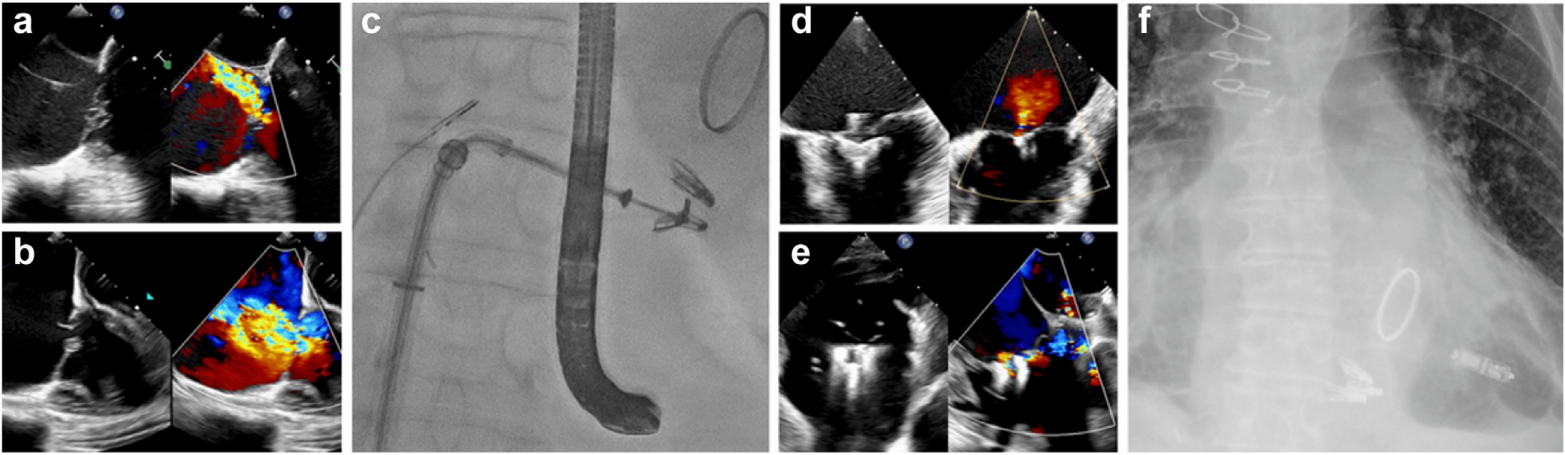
A case representative of the challenges presented with CIED and tricuspid regurgitation. A 91-year-old male with atrial fibrillation, a mechanical mitral valve, and a pacemaker with a single right ventricular lead developed severe tricuspid regurgitation and congestive hepatopathy-related cirrhosis. He was referred for lead extraction, placement of a leadless pacemaker, and ultimately TEER of the tricuspid valve with three MitraClip devices. (a) Pre-extraction TEE revealing severe TR. (b) Postextraction TEE revealing disrupted tricuspid leaflets and severe TR. (c) Fluoroscopy during the TEER procedure showing placement of the second clip under TEE and ICE guidance. (d) ICE imaging showing two tricuspid leaflets being captured by a MitraClip and ultimately trace TR. (e) Final TEE imaging showing the three MitraClips, pulling all three leaflets together, and the resulting trace TR. (f) Final chest X-ray showing the three MitraClips and the leadless pacemaker Abbreviations: CIED, cardiac implantable electronic device; ICE, intracardiac echocardiography; TEE, trans-esophageal echocardiography; TEER, transcatheter edge-to-edge repair; TR, tricuspid regurgitation.
